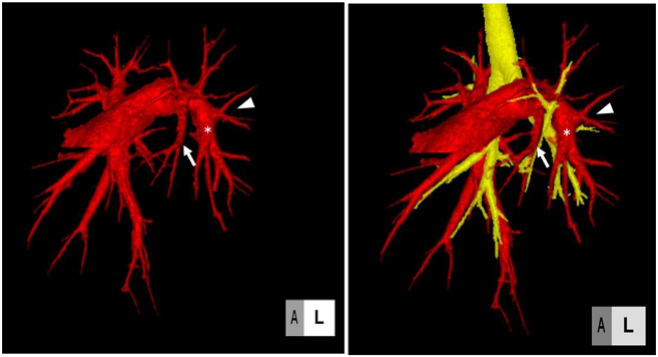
Three-dimensional computed tomography of the pulmonary artery, trachea and bronchus. Computed tomographic pulmonary angiography with three-dimensional reconstructions showing the left A4+5 artery (the mediastinal lingual artery) arising medial to A6. Red image, pulmonary artery; yellow image, trachea and bronchus; white arrow, A4+5 (the mediastinal lingual artery); white arrowhead, A6; asterisk, common basal artery.
